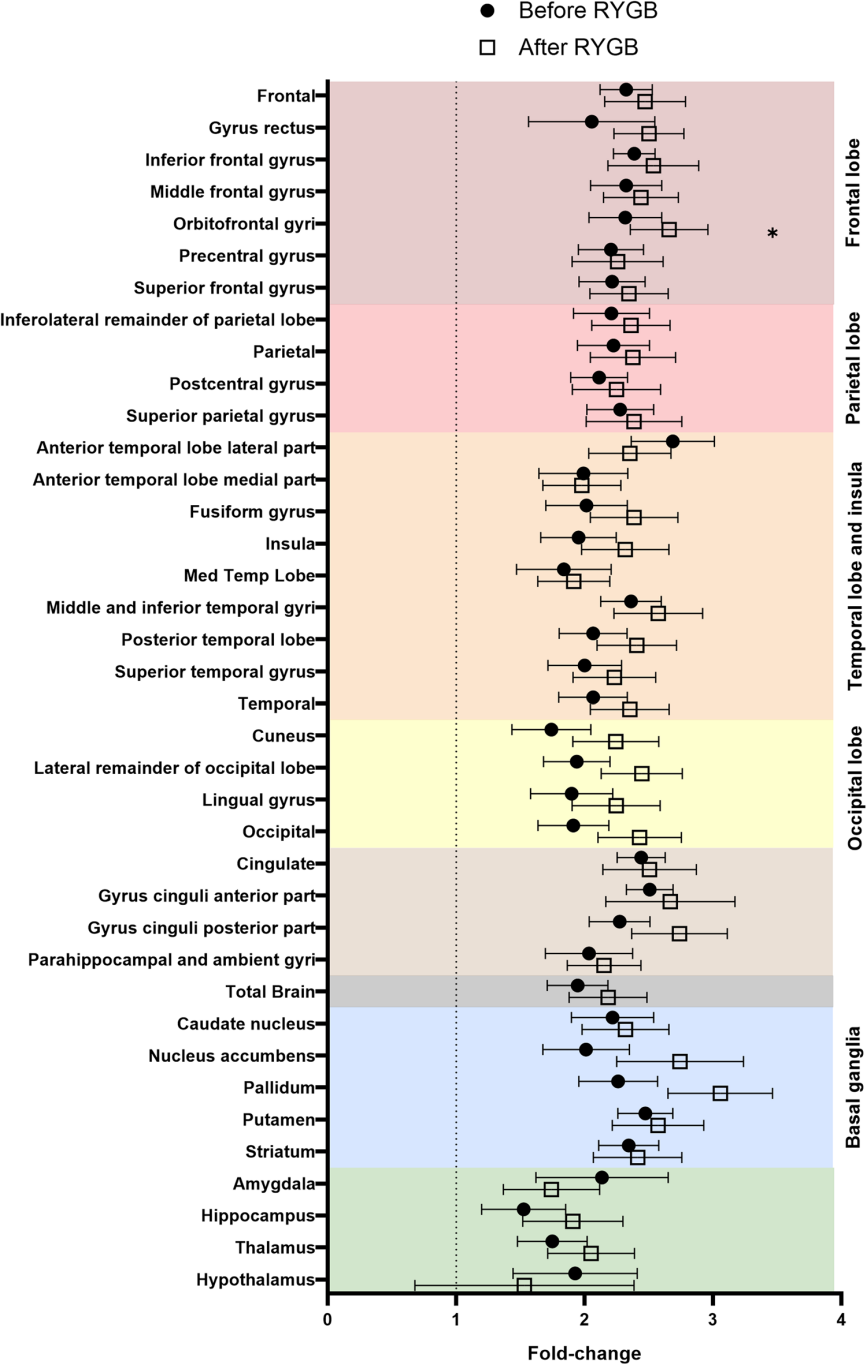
Fold-change (ratio) of regional FDG clearance during hypoglycemia vs normoglycemia before (filled circles) and after (open squares) RYGB. Paired t tests. p* < 0.05. Dots represent means and lines represent standard errors. Background colors represent the anatomical classification of the regions. Dark red: frontal cortex; bright red: parietal cortex; orange: temporal cortex; yellow: occipital cortex; brown: limbic cortex; black: whole brain; blue: basal ganglia; green: other subcortical nuclei
